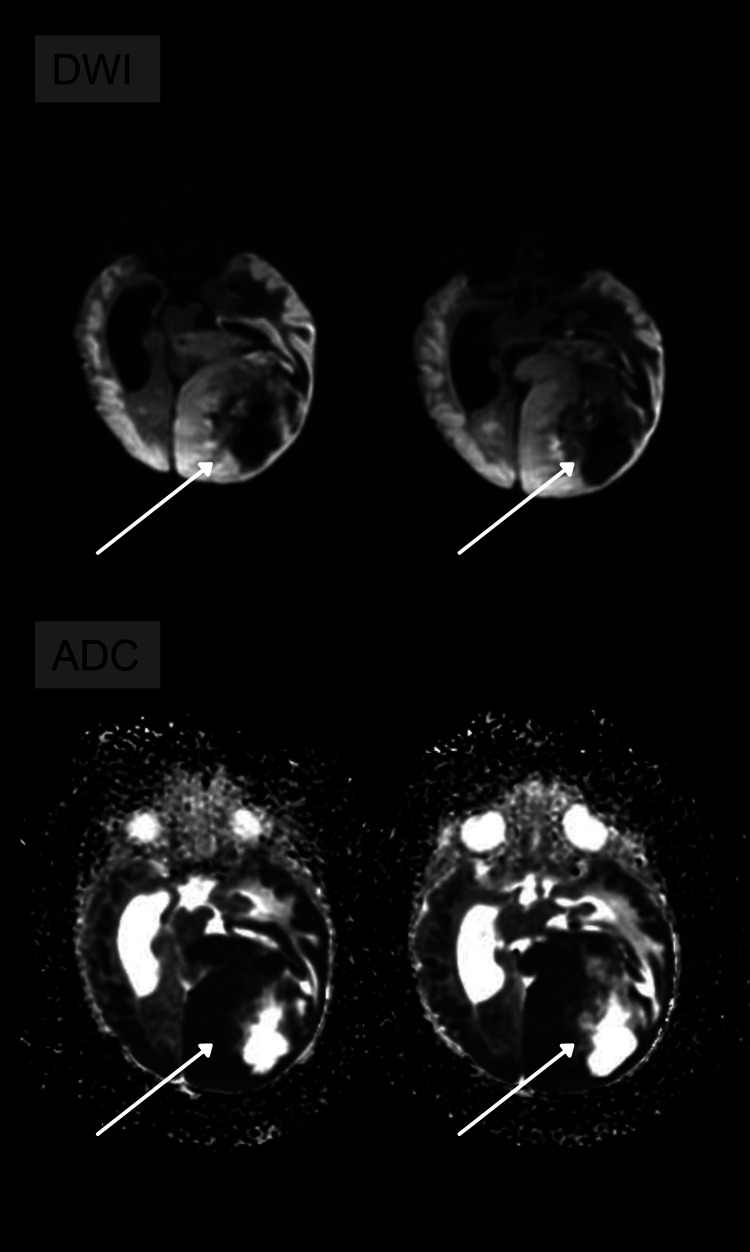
Heterogeneous areas of diffusion restriction are noted on the DWI and ADC map, indicating the high cellularity of the lesion. ADC: apparent diffusion coefficient; DWI: diffusion-weighted imaging
